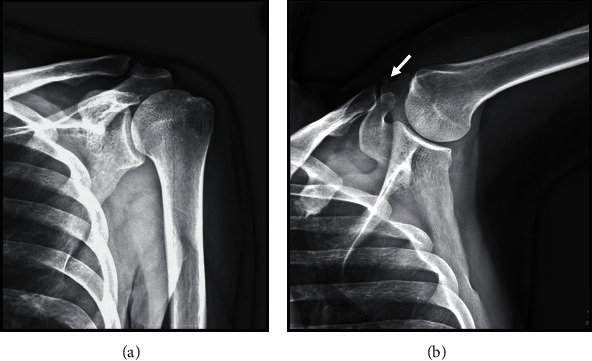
(a) Anteroposterior radiograph of the left shoulder showing a normal appearance. (b) Plain radiograph with the arm in an elevated position showing calcification around the anterior acromion. The arrow indicates the calcium deposit.
